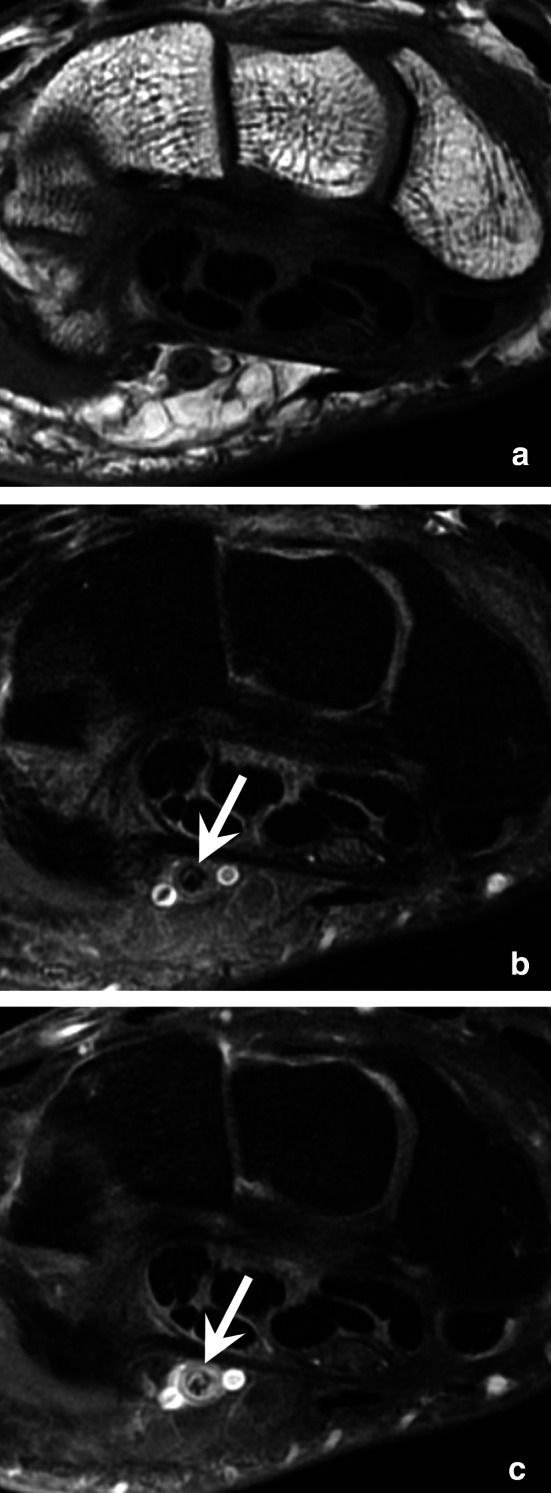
A pitfall in a case of HHS in a 45-year-old manual worker with an occlusion of the UA. a–c Axial T1-weighted (a) and T2-weighted (b) MR images and contrast-enhanced T1-weighted fat-suppressed sequence (c) showing a subtle thickening and parietal enhancement of the UA (arrow). The thrombus should not be confused with a flow phenomenon
